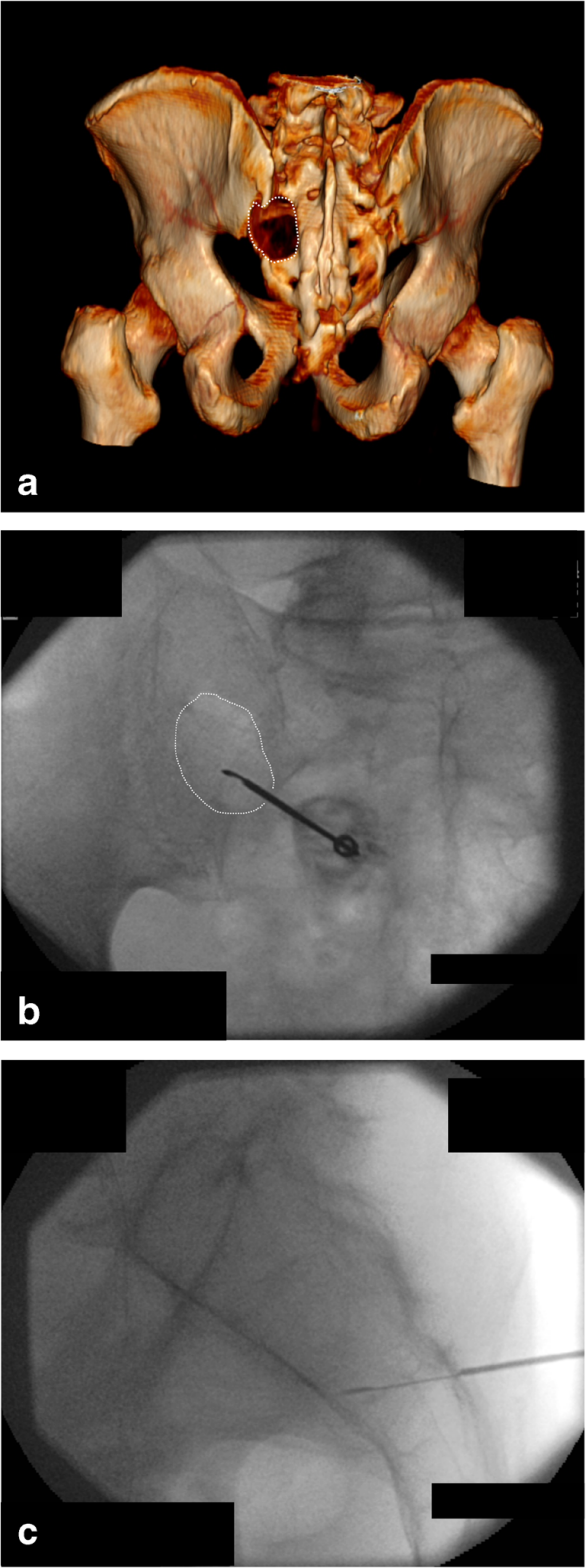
A 77-year-old man with clinical background of melanoma presenting with a new lytic lesion in the left sacral wing. a CT 3D reconstruction shows the lytic lesion (*white dotted line*). b, c PA and lateral fluoroscopic views were used to guide the biopsy, which was performed with a coaxial 16-G cutting needle and confirmed melanoma metastasis
